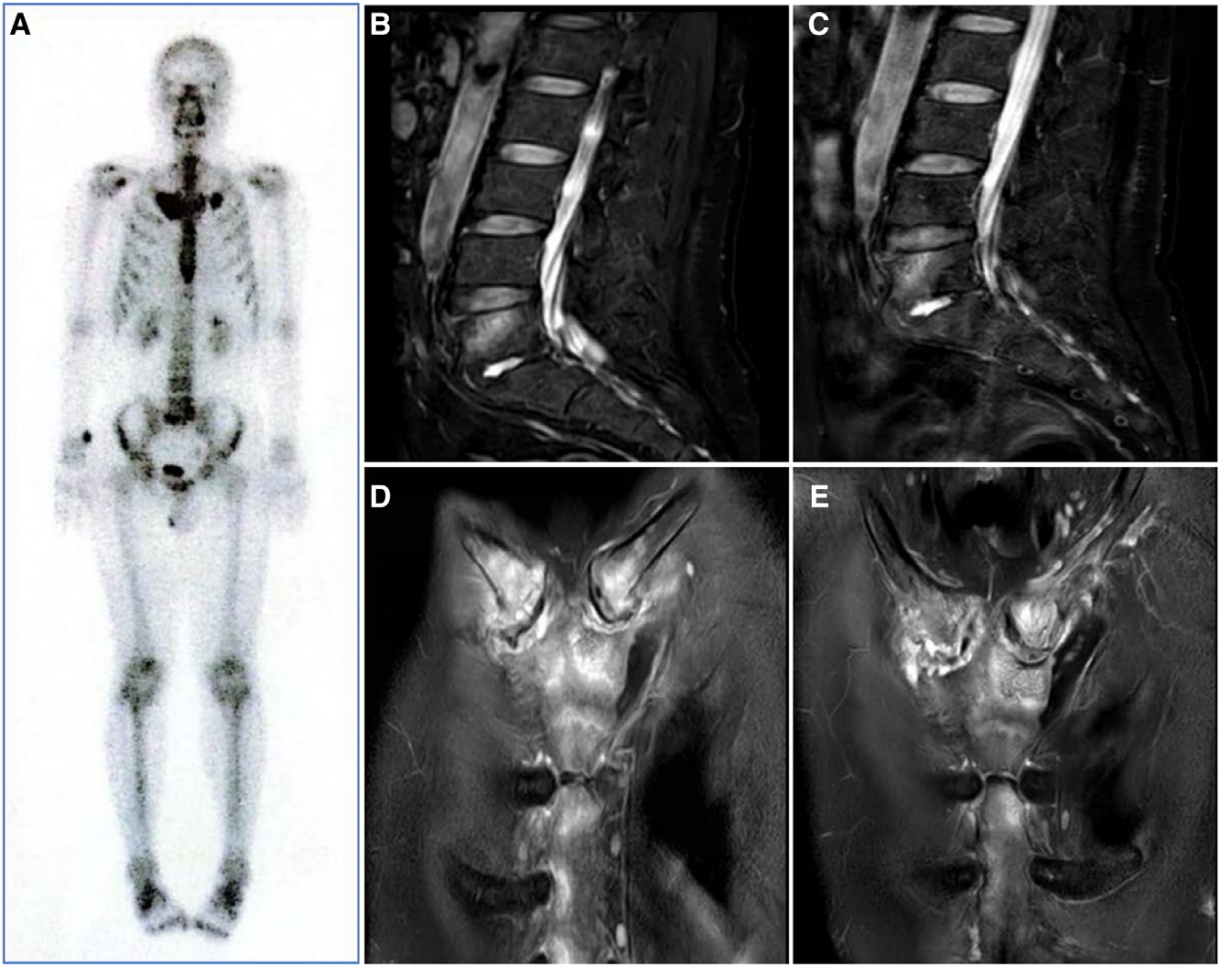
(A–E): Whole-body bone scan and magnetic resonance imaging of the whole spine of a 46-yr-old male who presented with the syndrome at week 0. Whole-body bone scan revealed good uptake and clear visualization of bones throughout the body, with areas of increased radioactivity uptake observed in the bilateral sternoclavicular joints and adjacent sternums, clavicles, lower sternums, right 8th rib proximal to the costovertebral joints, and the edges of the L5 and S1 vertebrae, while the remaining bones did not show any obvious abnormal areas of increased or decreased radioactivity. Sternoclavicular joint and Lumbar MRI suggested: Bilateral sternoclavicular and lumbar 5 vertebral bone marrow edema was less extensive and less intense than before.
